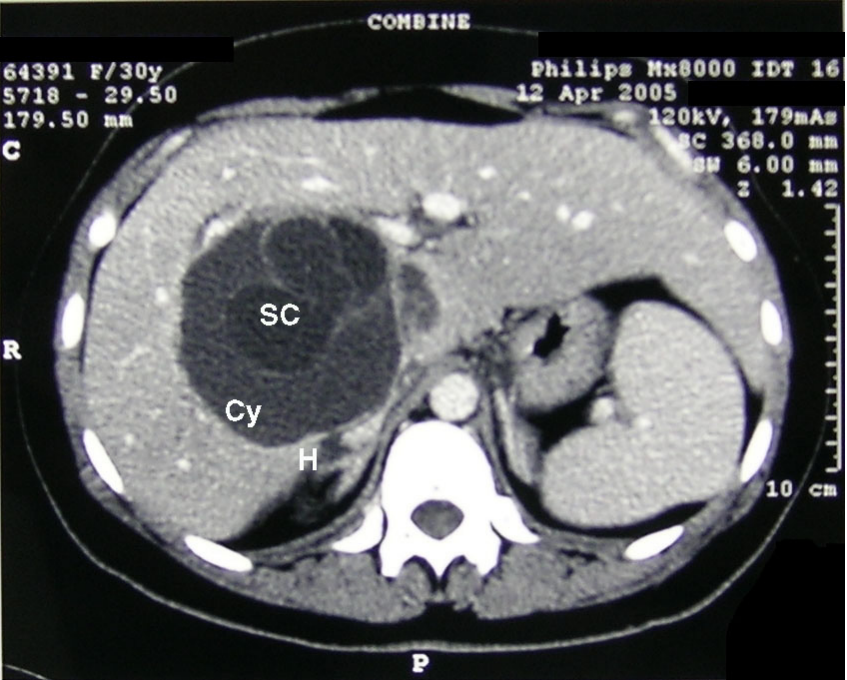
Contrast-enhanced CT scan. Cy: cyst; SC: small cysts; H: restricted hemorrhagic area adjacent to fissured cyst wall.
